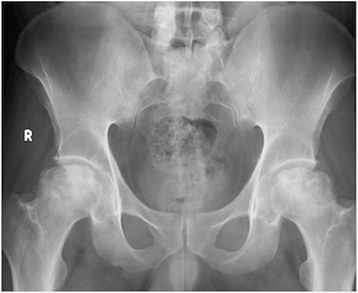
Preoperative X-ray. Anteroposterior pelvis X-ray demonstrating bilateral ON of the femoral head.
